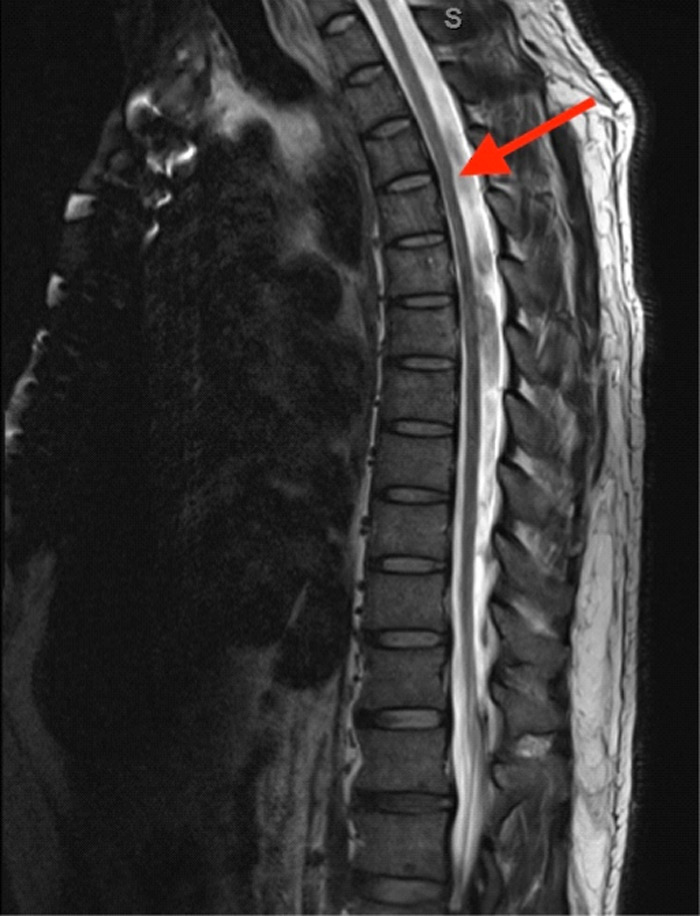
Magnetic resonance imaging with and without contrast showing focal anterior displacement of the thoracic spinal cord at the third and fourth thoracic levels (arrow). In the setting of a prominent dorsal subarachnoid space with altered cerebrospinal fluid flow dynamics, the findings demonstrate a dorsal arachnoid web.
